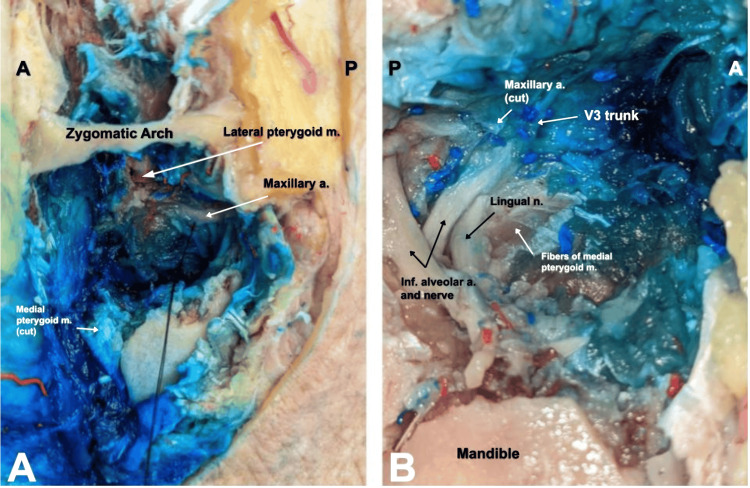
Methylene blue spread following ultrasound-guided injections in the lateral pterygoid and infratemporal planes. A) Spread of methylene blue after injection in the interfascial plane of the superior and inferior heads of the lateral pterygoid muscles. B) Spread of methylene blue after injection between the periosteum of the infratemporal fossa and the fascia of the lateral pterygoid muscles. a.: artery, m.: muscle, n.: nerve, Inf.: inferior, A: anterior, P: posterior.
